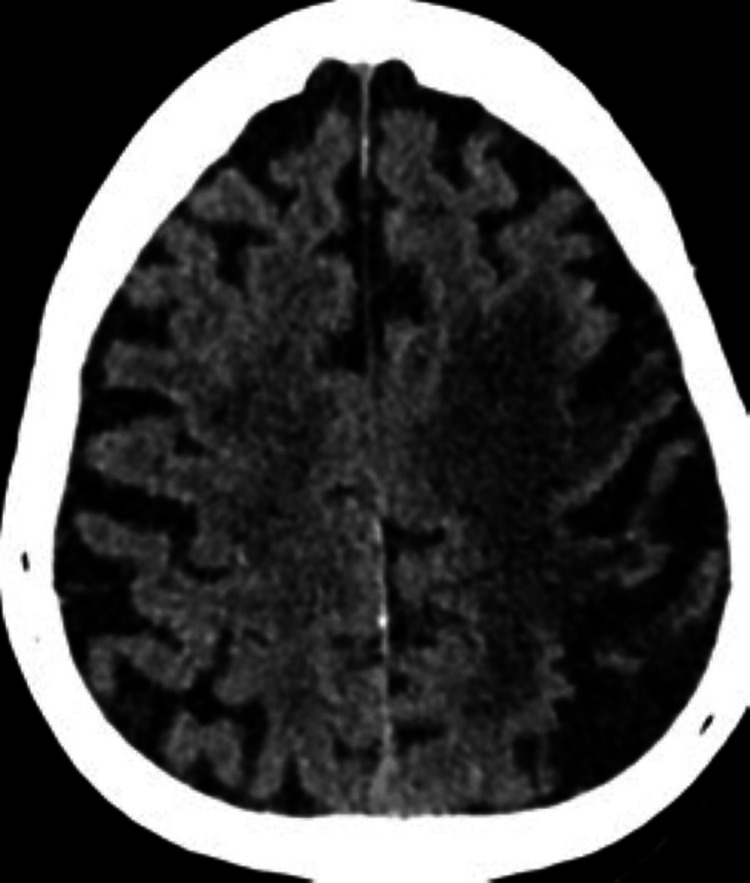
Brain CT of patient two The image shows chronic infarcts in the left superior frontal and parietal lobes with encephalomalacia.
